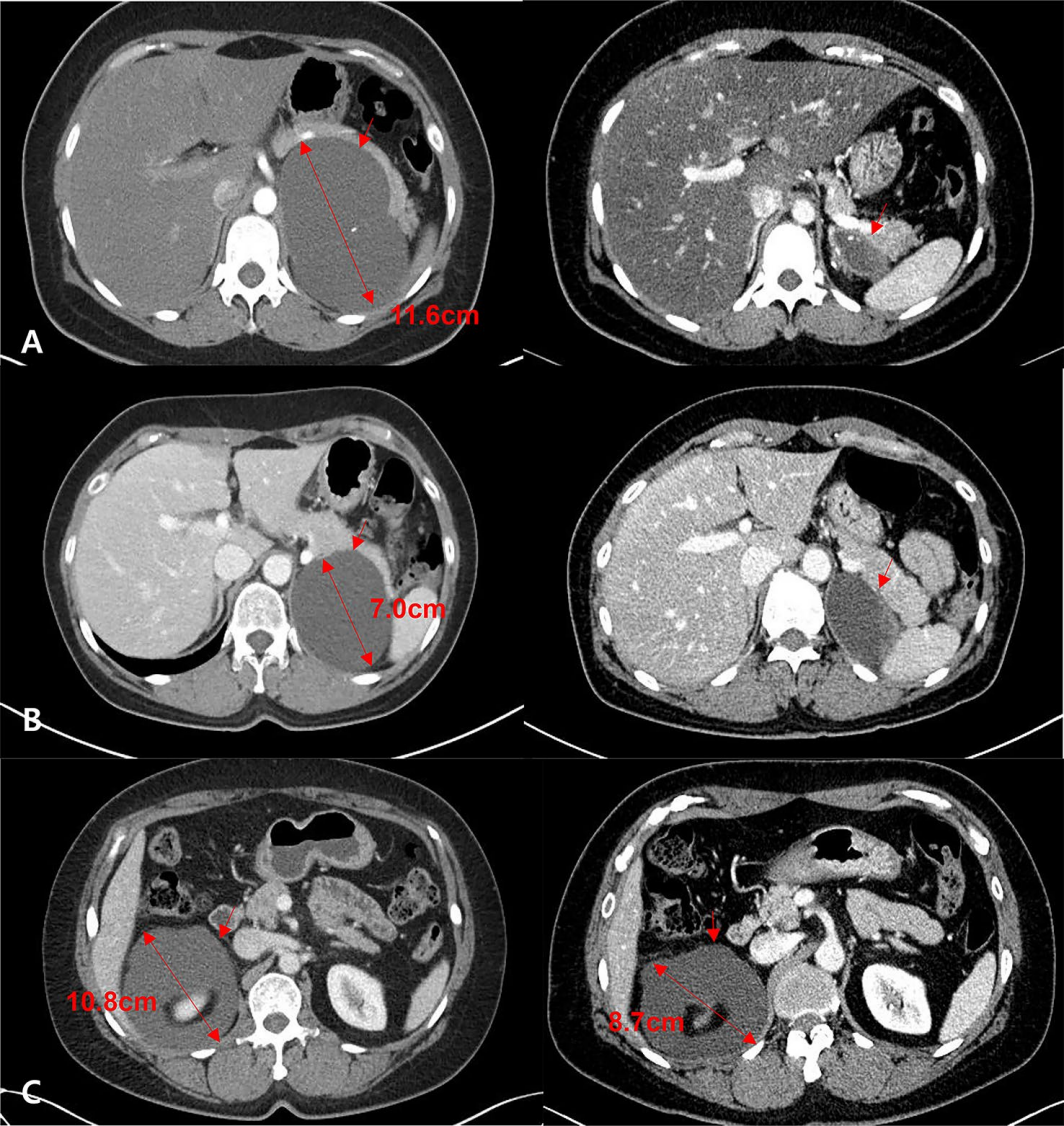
Axial computed tomography (CT) imaging of prior to treatment and 3 months after RFA of (A) Patient No. 3, who showed a good response, (B) Patient No. 9, who showed an intermediate response, and (C) Patient No. 1, who showed a poor response.
